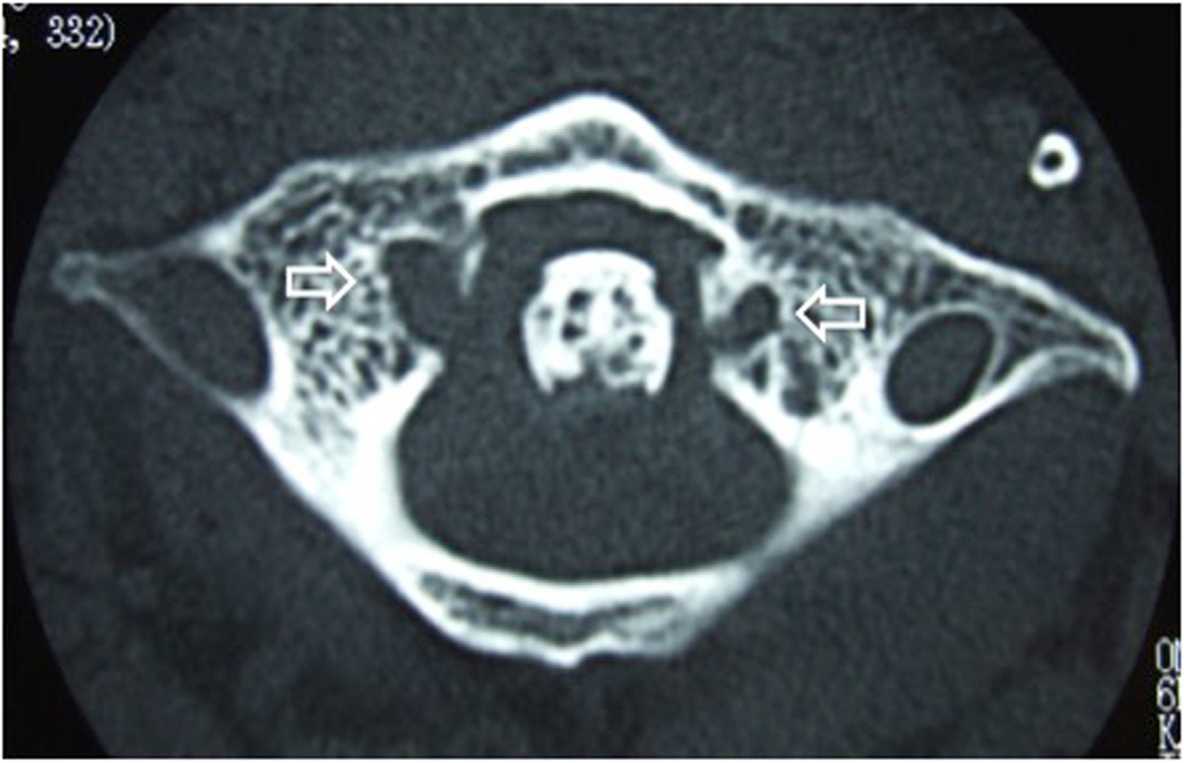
Computed tomography on admission. Erosive changes of the bilateral lateral masses of the atlas (*open arrows*) are visible
